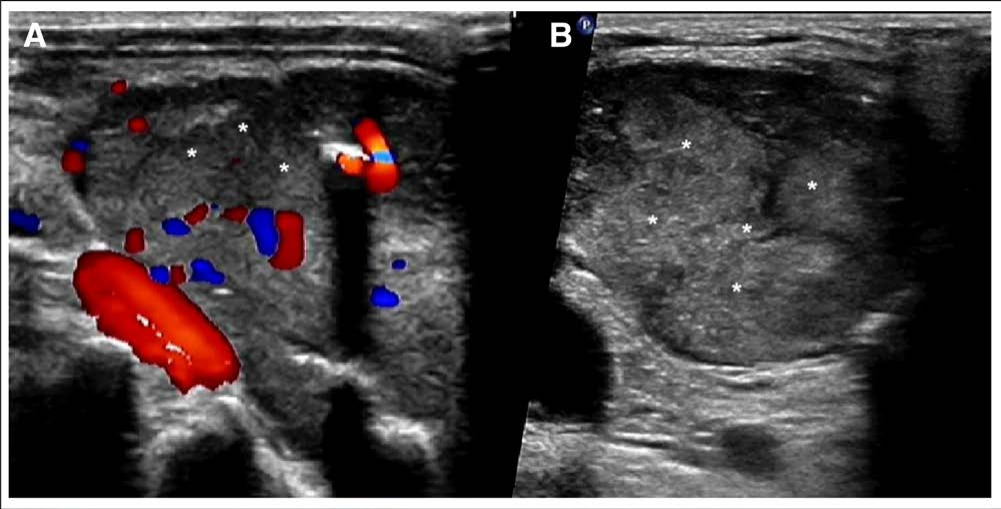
(A) and (B) The images of neck ultrasound showed this solid mass below the right lobe of the thyroid (White asterisks) with blood flow signals.
